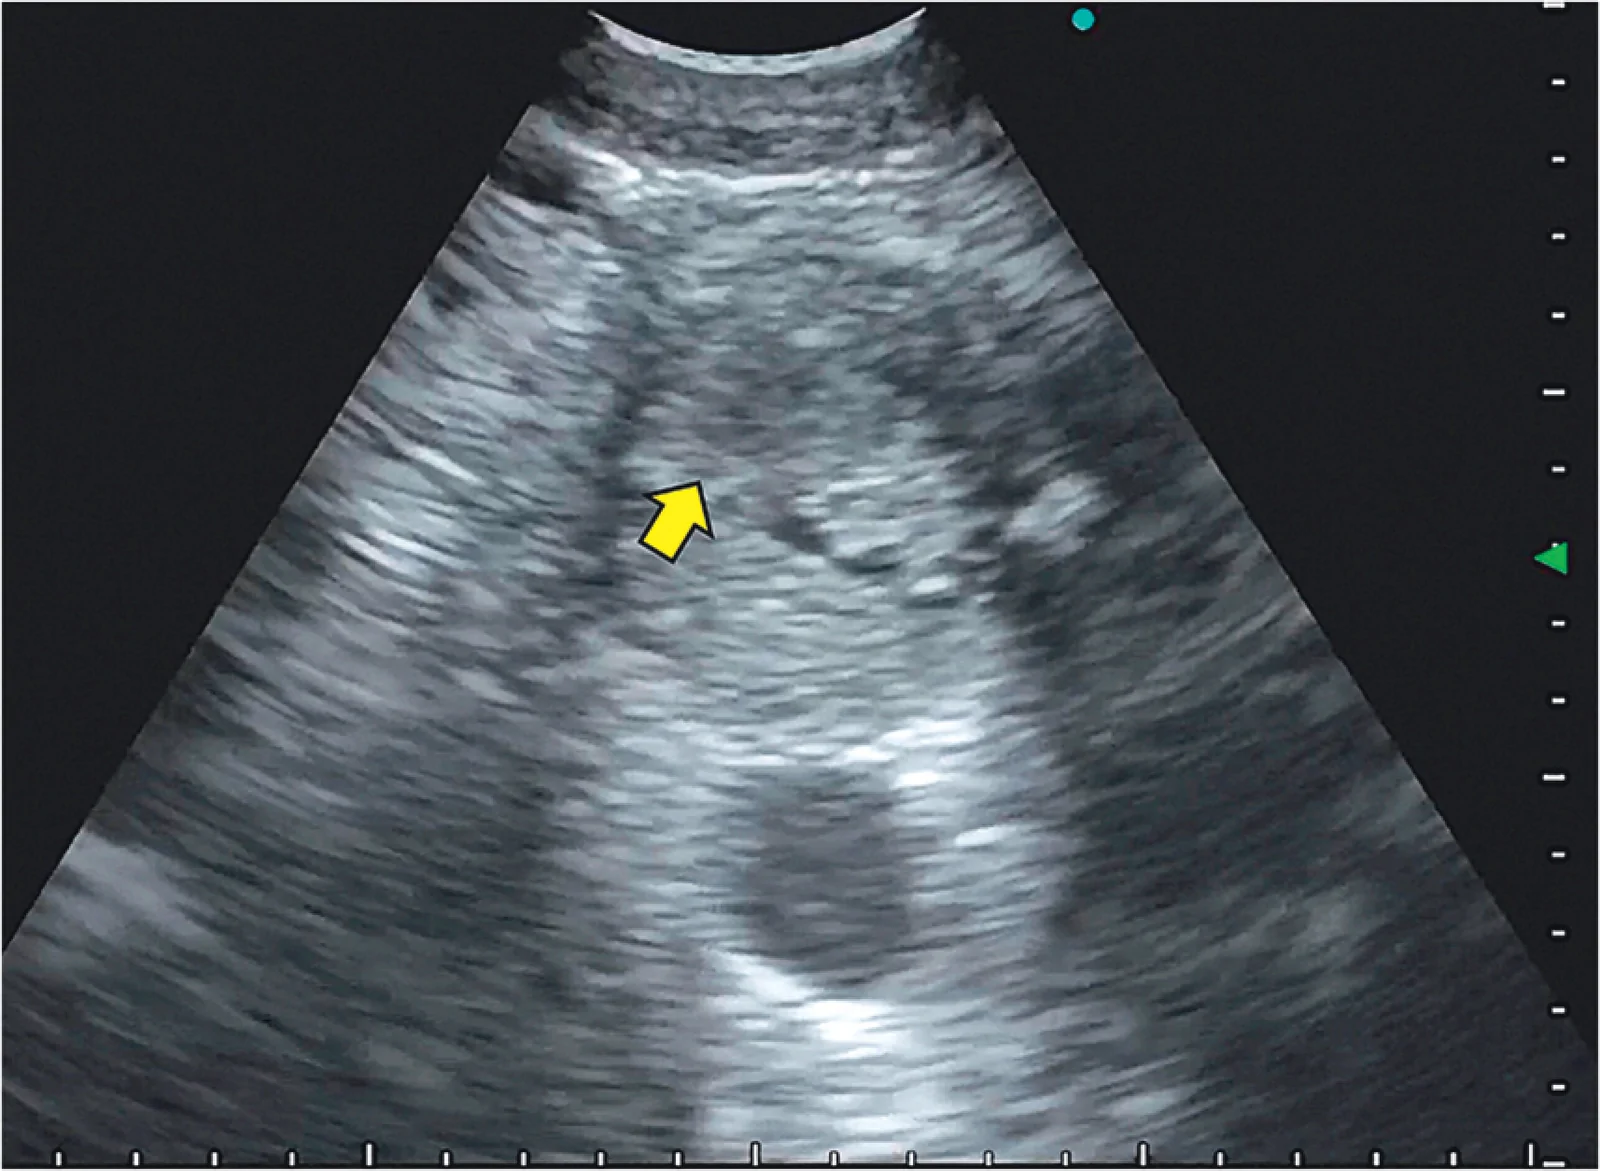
An endoscopic ultrasound image of the lesion. A
